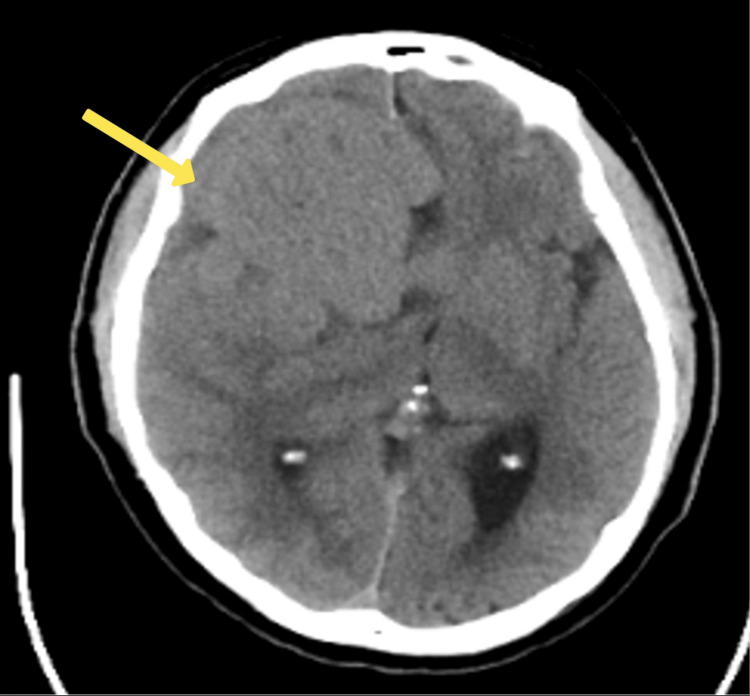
CT brain reveals a large mass (measuring approximately 6.6 x 4.7 x 6.5 cm) in the anterior cranial fossa on the right side (with associated mass effect and perilesional edema), causing erosion of the right anterior clinoid process and the roof and lateral wall of the right sphenoid sinus (see arrow). The superomedial wall of the apex of the right orbit is also eroded.
